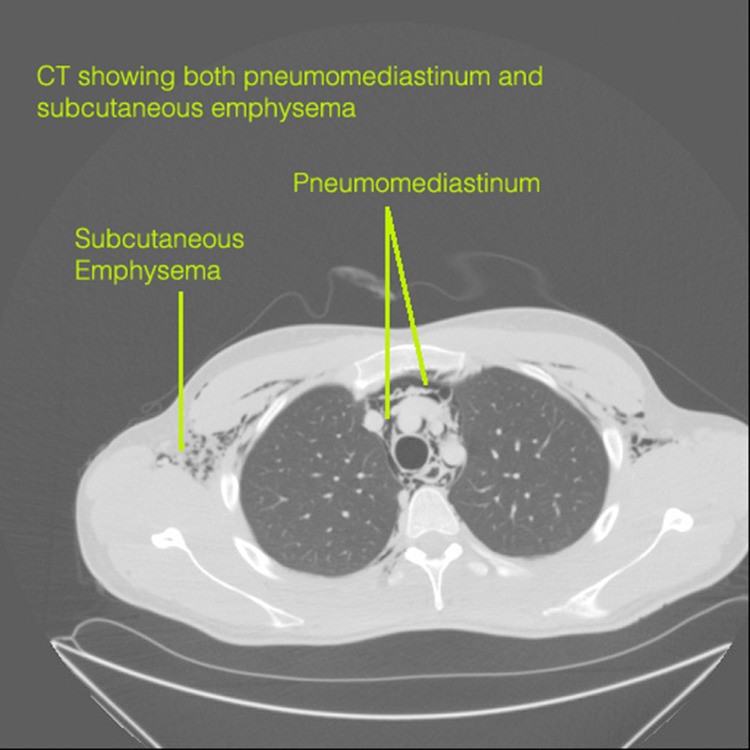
Computed tomography demonstrating pneumomediastinum and subcutaneous emphysema
